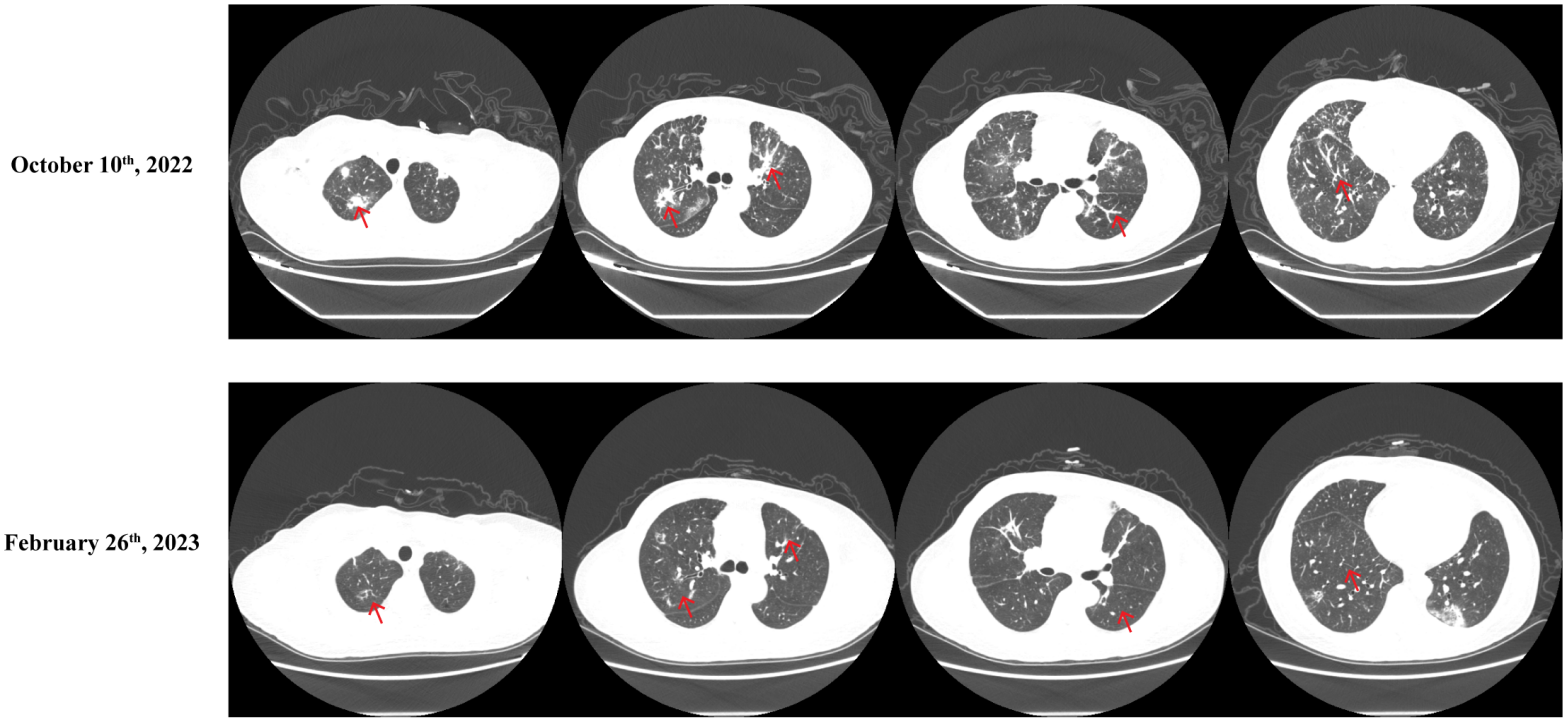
Comparative Chest CT Scans Conducted Before and After the Fifth DS8201 and Tislelizumab Treatment Regimen Cycle. Before: October 10, 2022, After: February 26, 2023.
